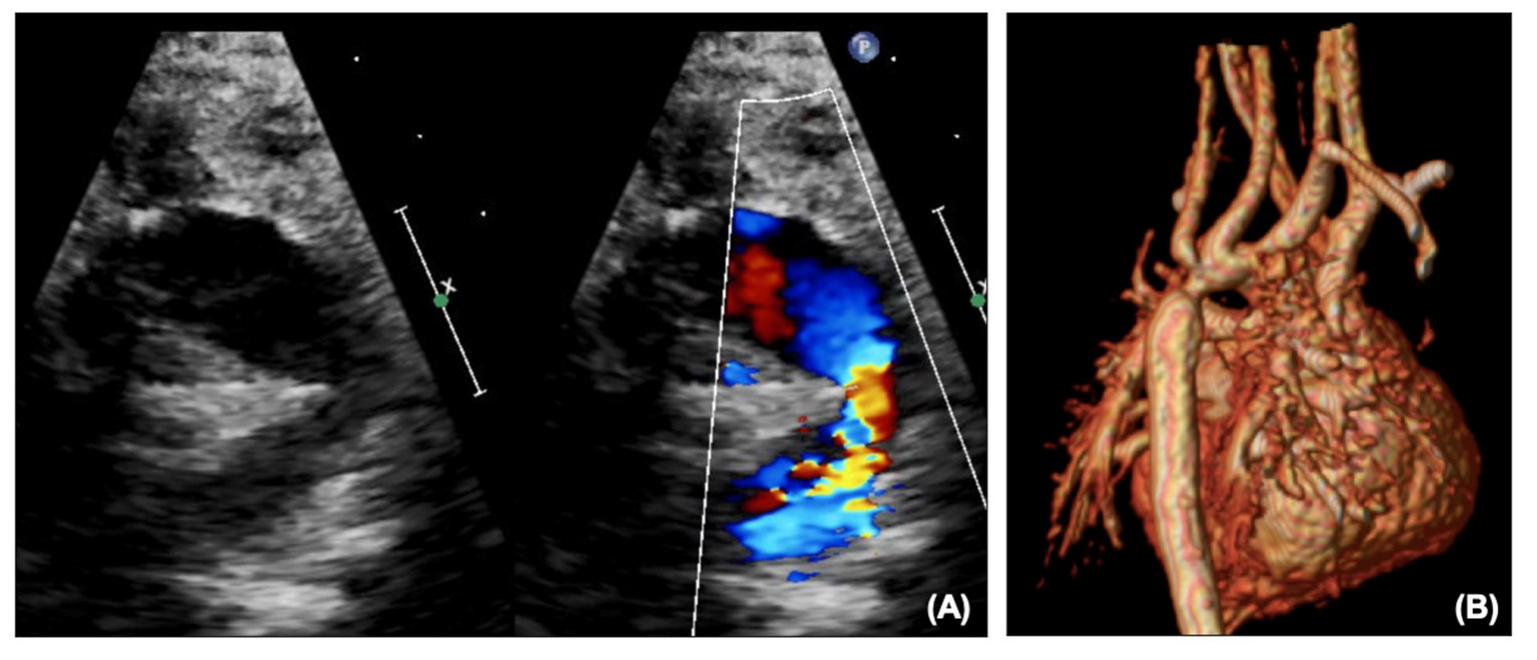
(A) Arch obstruction on echocardiography shown on 2D and color. (B) Arch obstruction on MRI.
